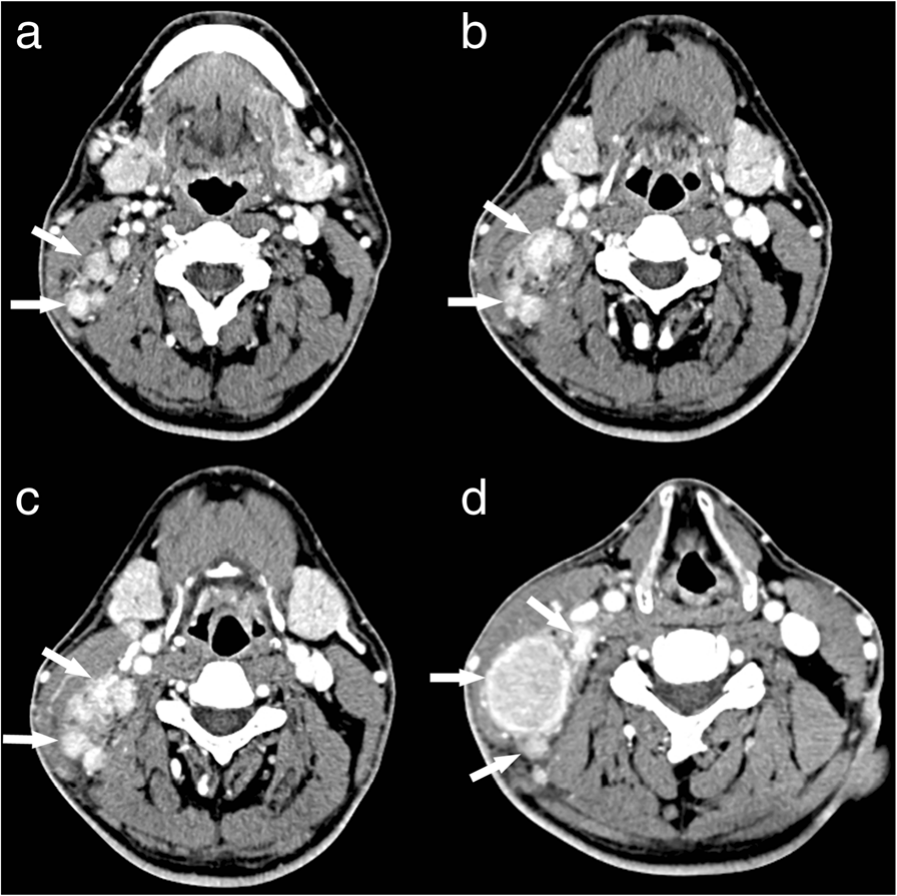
A 37-year-old man who presented with hyaline vascular type Castleman disease. a-d Contrast-enhanced CT images of the neck show multiple nodules with a marked enhancement pattern (white arrows), with all of the nodules located on the right side of the neck. There are no enlarged lymph nodes on the left side of the neck
